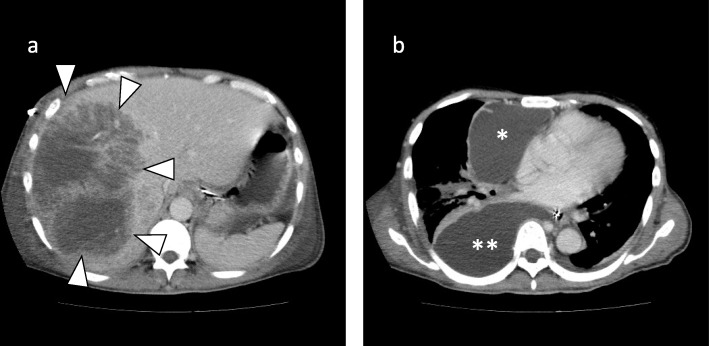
a CT showing a liver abscess in the right liver lobe partially containing necrotic solid tissue. b The liquid abscess cavities in the mediastinum (*) and right thoracic cavity (). CT: Computed tomography
